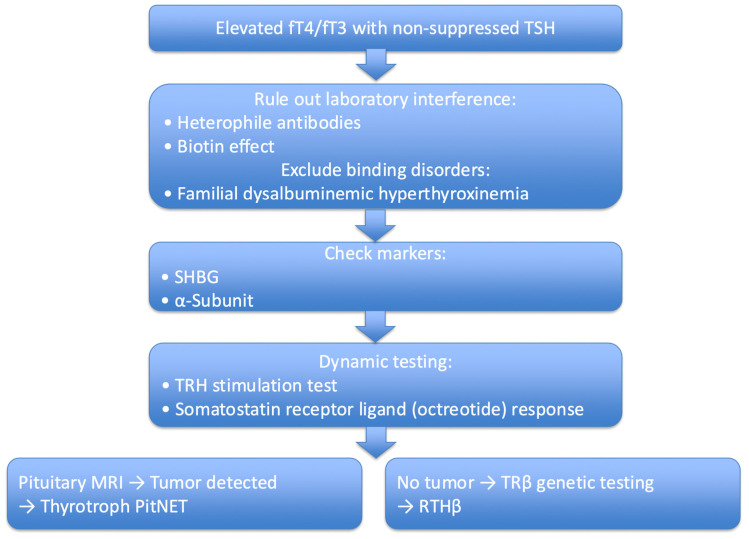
Stepwise diagnostic algorithm for inappropriate TSH secretion. The evaluation begins with confirmation of elevated fT4/fT3 levels in the presence of non-suppressed TSH. Laboratory interference and thyroid hormone binding abnormalities should be excluded before proceeding to dynamic testing (TRH stimulation test and somatostatin receptor ligand response), MRI, and genetic analysis. Somatostatin receptor ligand response may provide supportive evidence but is not diagnostic in isolation. Measurement of the glycoprotein hormone α-subunit or sex hormone–binding globulin (SHBG) may provide supportive evidence. TRβ, thyroid hormone receptor β; RTHβ, resistance to thyroid hormone β.
